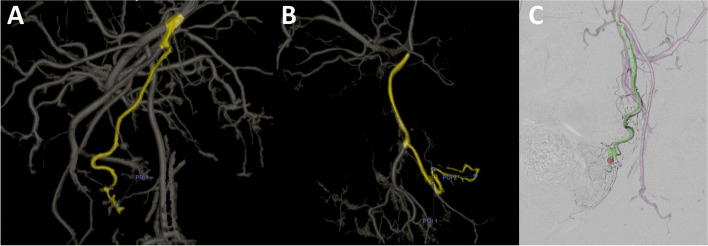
Virtual injection technology using GE Allia™ IGS 7 system. A 3D reconstruction after obtaining cone beam CT from internal iliac artery (2 mL/sec, total volume 24 mL, acquisition at 6 s delay), and then angulation was adjusted to best visualize the prostatic artery (POI = point of interest). B Virtual injection in another patient, with removal of the osseous structures to assist the visualization and identification of the prostatic artery. C 3D overlay can also be performed on live fluoroscopy to guide and facilitate the catheterization
